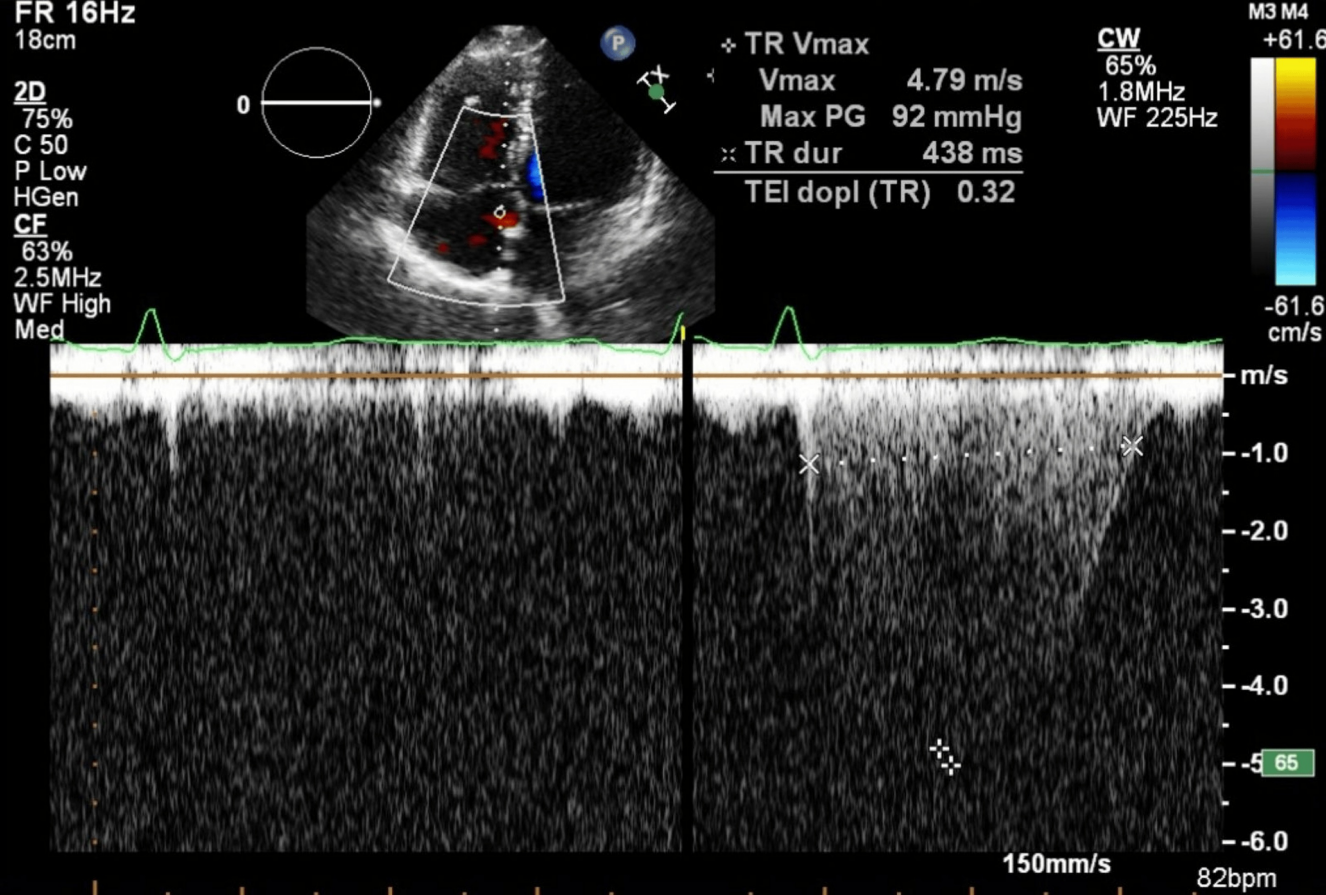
Apical four-chamber view with color Doppler demonstrating a TR jet, with CW Doppler aligned through the regurgitant flow CW: continuous wave; TR: tricuspid regurgitation The CW Doppler spectrum shows a peak TR velocity (Vmax) of 4.79 m/s, corresponding to a calculated peak systolic transtricuspid pressure gradient of 92 mmHg (modified Bernoulli equation). Tricuspid regurgitation duration is 438 ms, and the Doppler-derived Tei index from the TR signal is 0.32, indicating preserved to mildly impaired global right ventricular function despite markedly elevated right ventricular systolic pressure, consistent with compensated pressure overload. Measurements were obtained from an incomplete jet due to a limited acoustic window and represent the best achievable measurement and should be interpreted as an estimated value
